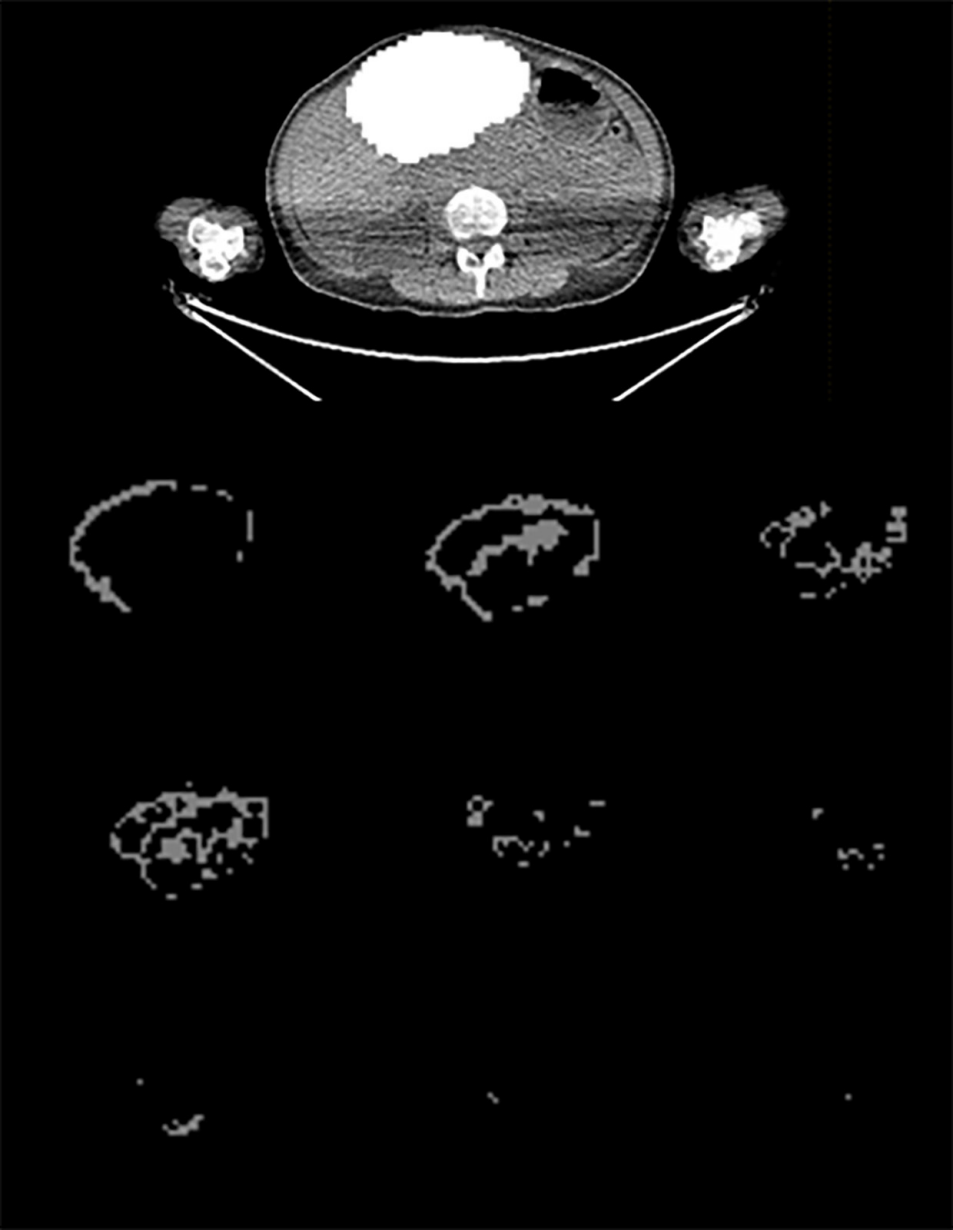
Axial CT image showing a slice of the main VOI at the level of the liver (top image) and the nine derived VOIs (bottom images) based on the SUV thresholds for the patient presented in Figures 1 and 2.
